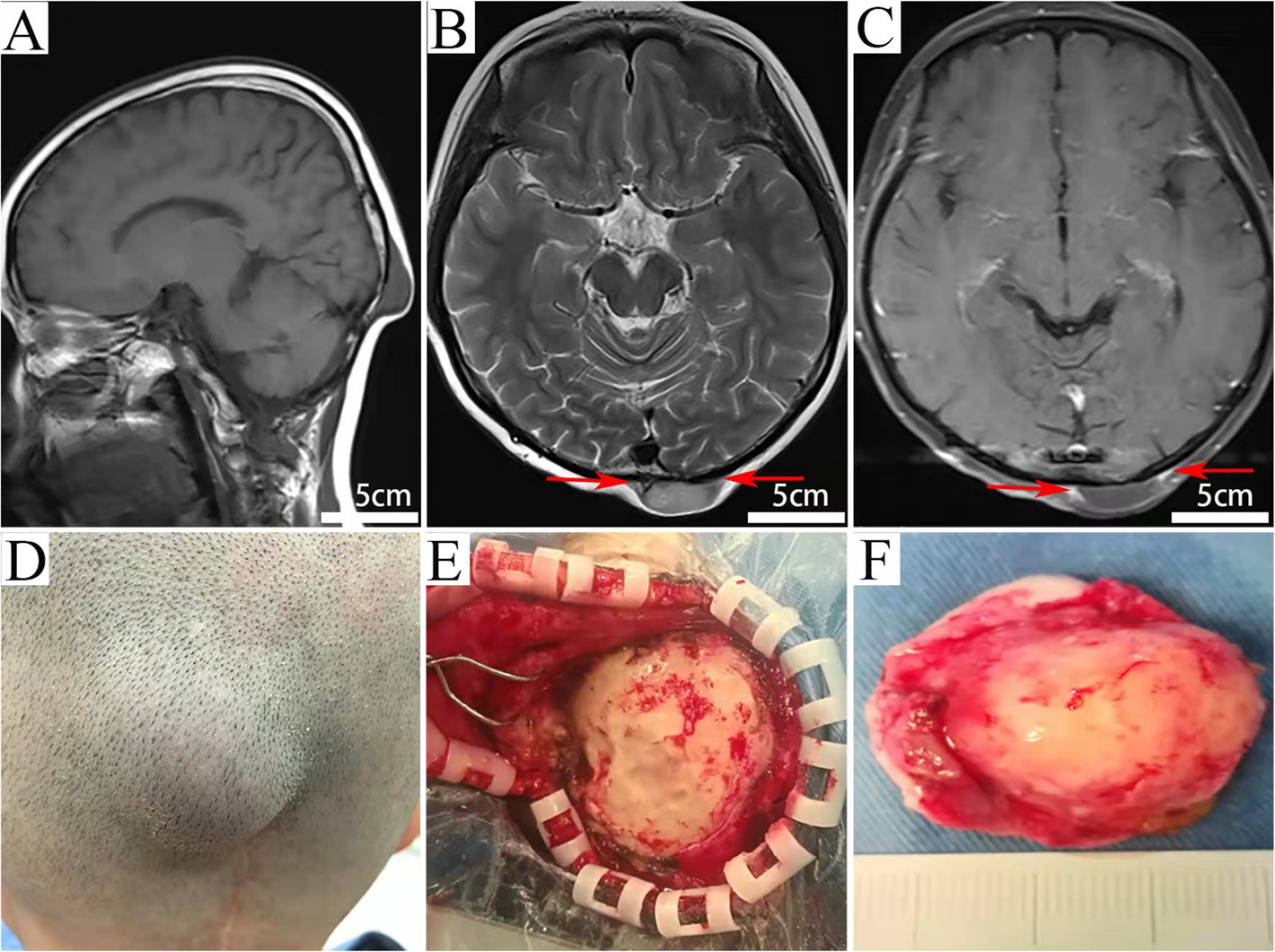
T1-weighted image (A), T2-weighted image (B), and contrast-enhanced MRI scans (C) reveal a lesion with well-defined borders under the left occipital scalp. It exhibits hypointensity on the T1-W sequence image (A), slightly hyperintensity on the T2-W axial image (B) and mild peripheral enhancement after contrast administration (C). “Tail sign” is found on T2-W axial image (B, red arrows), and is more obvious in the Post-contrast images (C, red arrows); Intraoperative photographs show the skull was compressed and deformed by the tumor (E). The tumor is grayish and about 35 × 25 cm in size (F)
